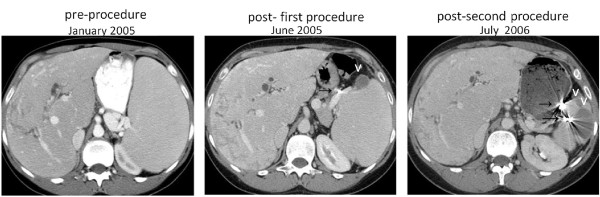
Patient 2, serial axial CT scans of the abdomen, demonstrating a heterogeneous liver, radiopaque material placed in the branches of the splenic artery (→), which has resulted in wedge shaped splenic infarctions (v). The splenomegaly is seen to progressively resolve, and there is progressively less impingement upon the left kidney.
